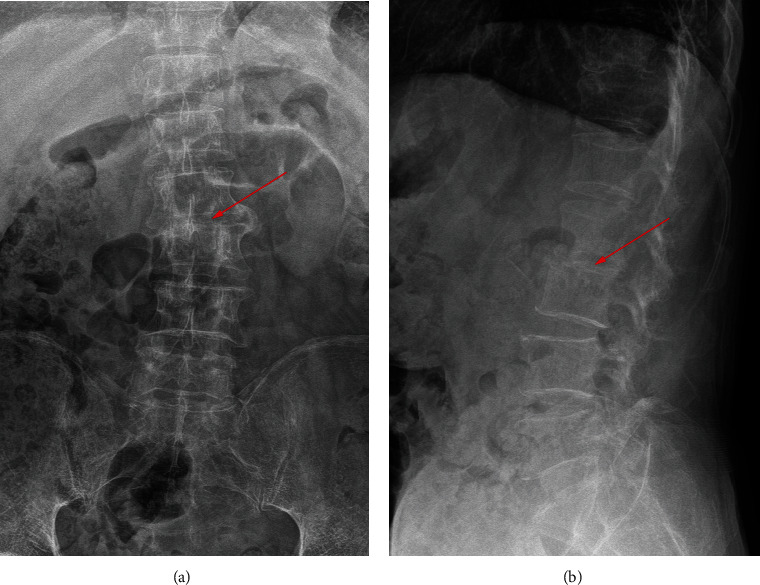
X-ray examination of a patient with spinal tuberculosis. The arrow points to the location of the lesion. (a) X-ray image in posterior-anterior position. (b) X-ray image in lateral position.
